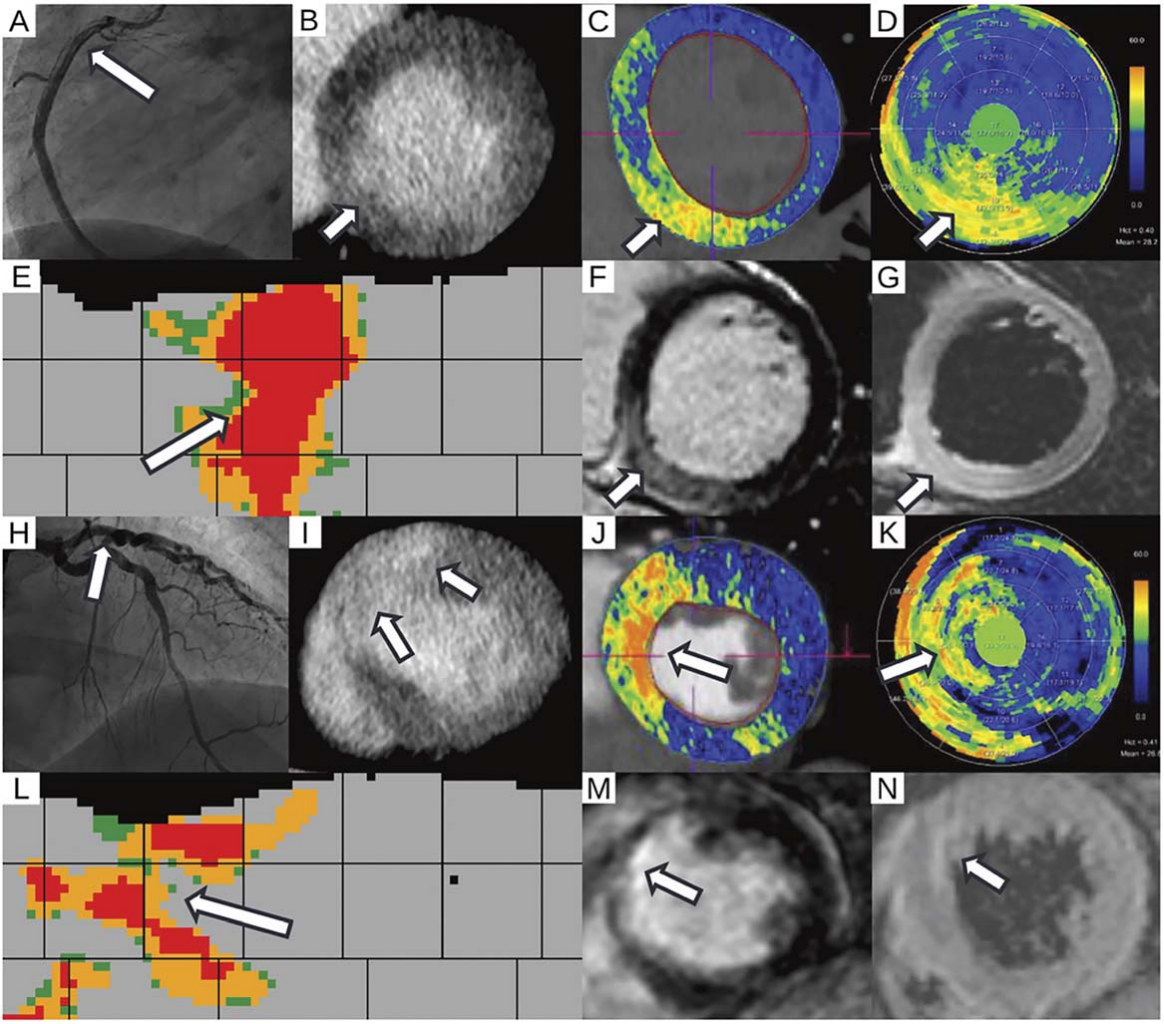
Myocardial injury with and without edema in spontaneous coronary artery dissection. A–G, A 62-year-old female (*patient 2*) with (A) dissection of the proximal right coronary artery in invasive coronary angiography (arrow). (B) Acute phase late enhancement CT performed 3 days after symptom onset revealed a large inferoseptal infarct, also seen in (C) overlay images, (D) polar, and on (E) atlas maps highlighting ECV distribution with noise-suppressed segmental visualization (arrows). F, Late gadolinium enhancement cardiac MRI performed on the same day as acute phase LE CT confirmed a smaller inferoseptal infarct with edema on (G) T2-weighted cardiac MRI (arrows), indicating partial concordance between CT and cardiac MRI. The larger infarct size in CT could be explained by edema in the acute phase. H–N, A 53-year-old female (*patient 8*) with (H) dissection of the mid left anterior descending artery in invasive coronary angiography (arrow). I, Follow-up CT 116 days after symptom onset revealed an anteroseptal infarct also seen in (J) overlay images, on (K) polar, and on (L) atlas maps depicting filtered segmental ECV elevations. M, Late gadolinium enhancement cardiac MRI performed one the same day as follow-up LE CT depicted the corresponding infarct without edema on (N) T2-weighted cardiac MRI (arrows), indicating total concordance between CT and cardiac MRI.
